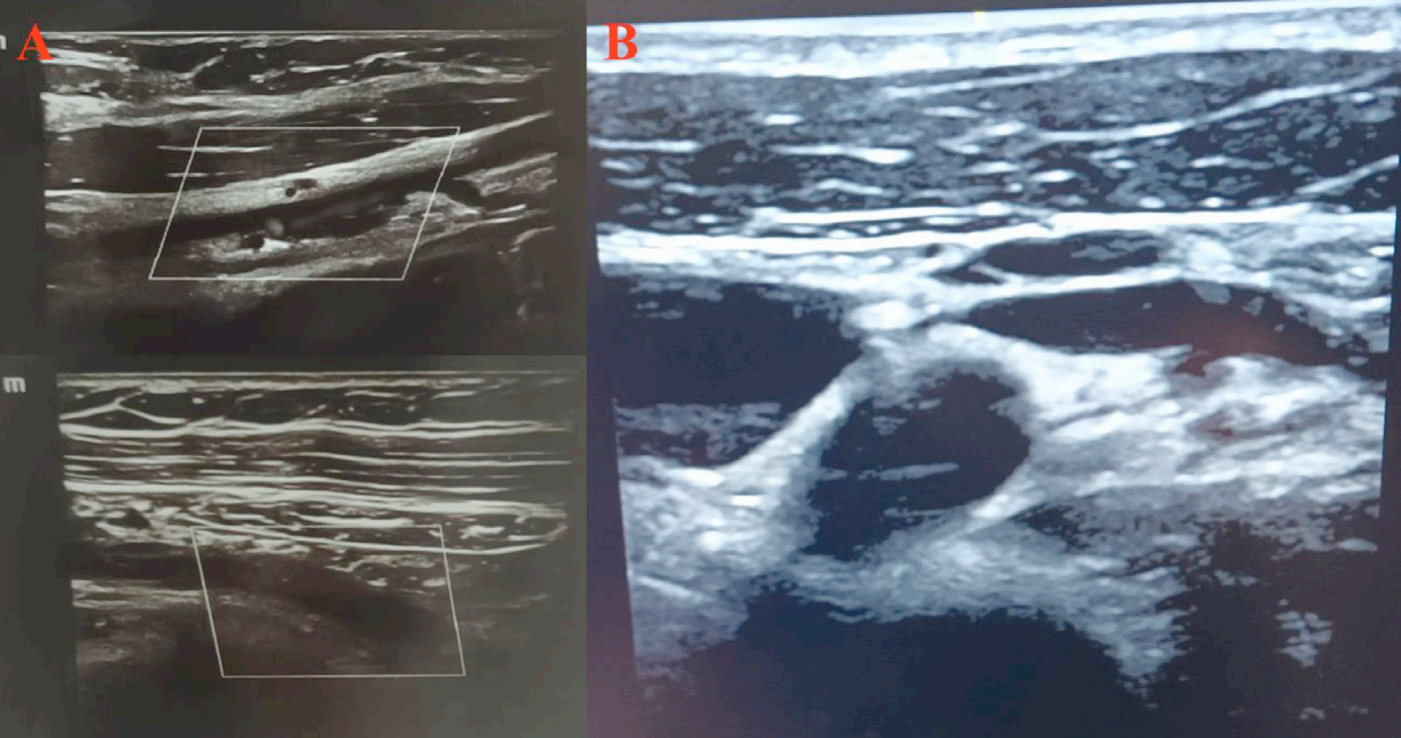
Color Doppler ultrasound (A) revealing subacute thrombosis in the distal subclavian vein and the upper third of the axillary vein, extending to the junction with the subclavian vein and (B) After maintaining apixaban treatment for eleven months.
